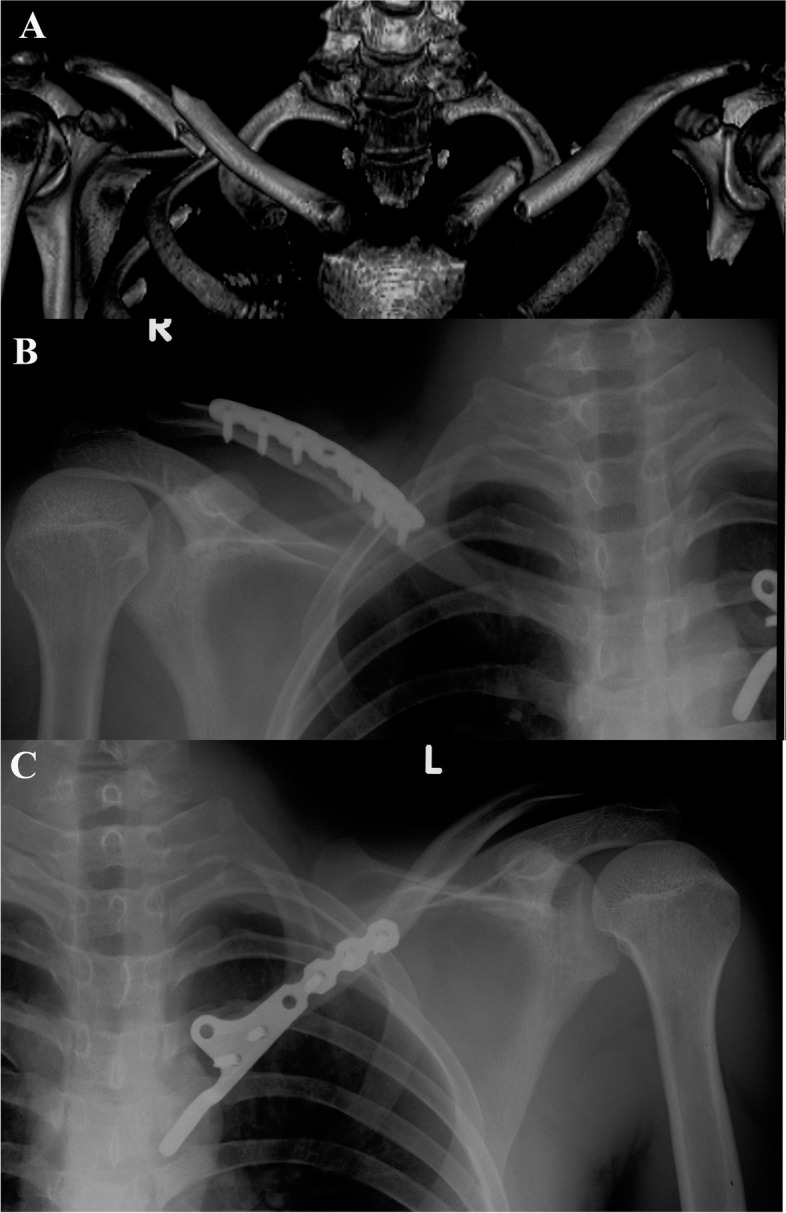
A Three-dimensional computed tomography reconstruction showed a displaced right middle-shaft clavicle fracture and a displaced left medial clavicle fracture. Both fractures were repaired by internal plate fixation. B, C An immediate postoperative radiograph showed anatomical reduction of both fractures as well as satisfactory positioning of the hook plate
